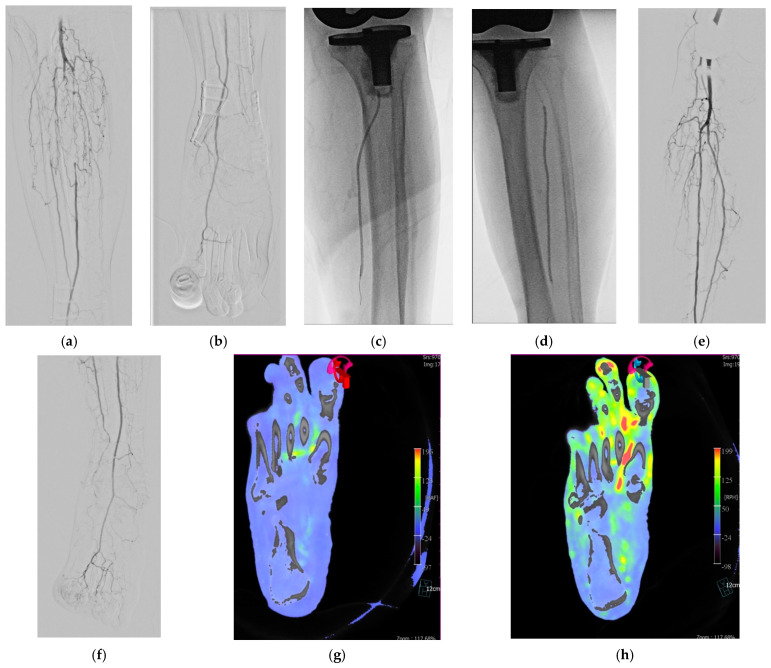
A 73-year-old woman with peripheral arterial disease underwent percutaneous transluminal angioplasty for the first and second toe wound. (a,b) Initial angiography shows long segmental occlusion of left anterior tibial artery (ATA) and posterior tibial artery (PTA) and total occlusion of left peroneal artery with decreased flow to the foot including first and second toe. (c,d) Recanalization with 0.014-inch guidewire and balloon angioplasty was done with a 2 mm–15 cm balloon catheter. (e,f) Completion angiography shows patent left ATA and PTA and increased flow to the foot especially first and second toe. (g,h) Cone-beam computed tomography-based foot perfusion mapping before (g) and after (h) angioplasty clearly shows markedly increased perfusion of the foot, especially first and second toe.
